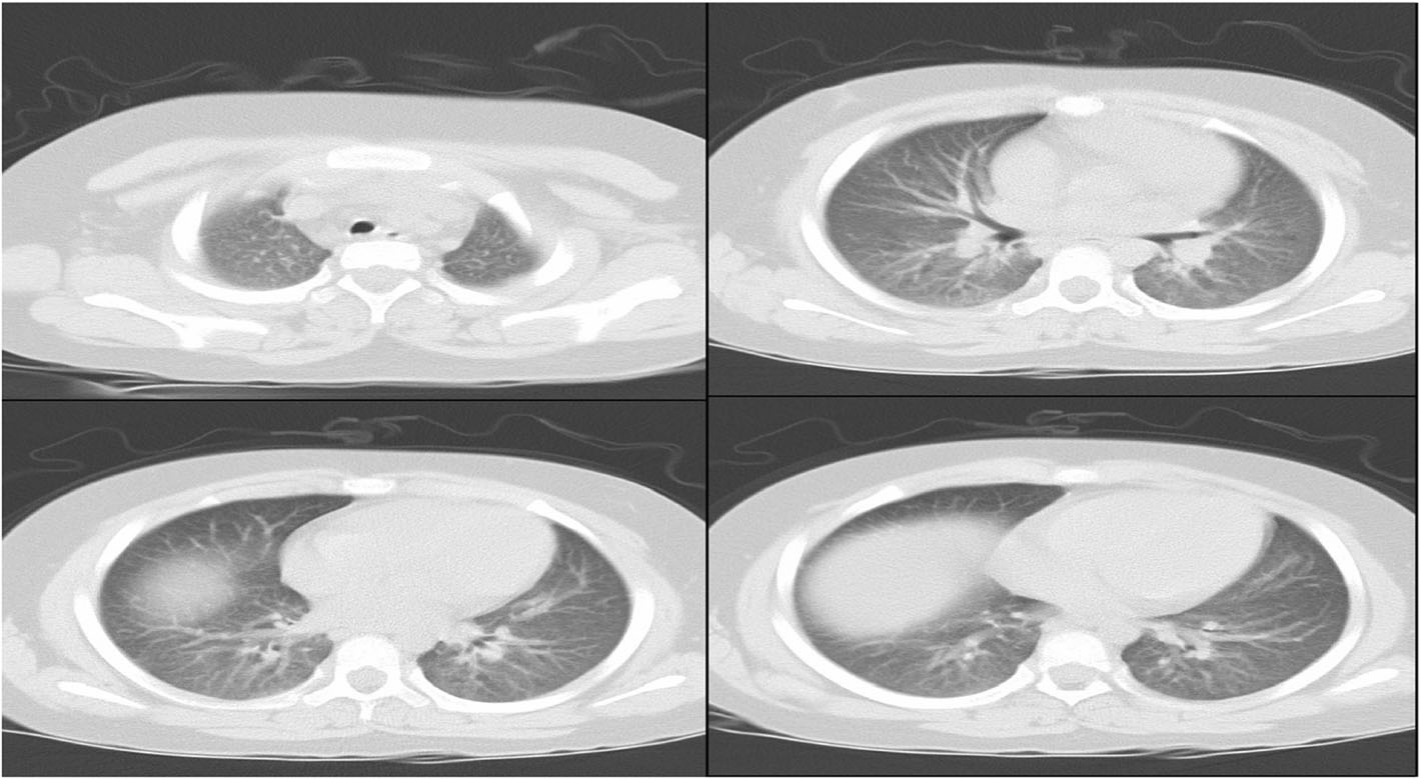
Chest CT of 30th March (Before the operation). This CT examination showed all lung fields were clean
